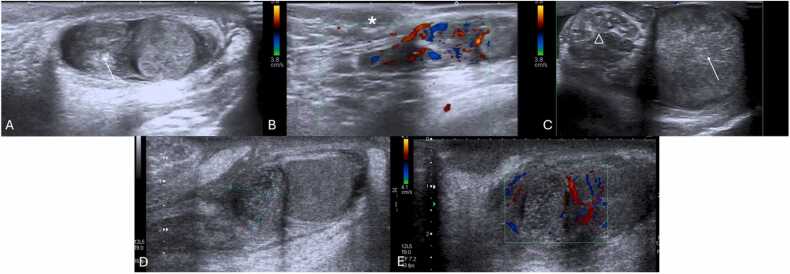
(A and B) Testicular ultrasound of a 6-year-old male with subacute scrotal pain. B-mode and color-Doppler ultrasound revealed an enlarged epididymis (arrow in A) with increased vascular flow on Color Doppler, allowing the diagnostic suspicion of epididymitis to be confirmed. Compare this with the scrotal color Doppler US transverse image of another 9-year-old male with acute, intense testicular pain. Notice the presence of enlarged epididymis (△ in C) and testicle (arrow in C) with absent flow, indicative of testicular torsion. (D and E) Testicular sagittal and transverse plane b-mode and color Doppler US images of a 12-year-old male with acute onset of testicular pain. On physical examination, a blue nodule adjacent to the painful area was present (no images available). US revealed a round, heterogeneous, avascular extra-testicular structure (between calipers in D), with preserved testicular vascular flow, allowing confirmation of the clinical suspicion of diagnosis of a torsed appendage.
